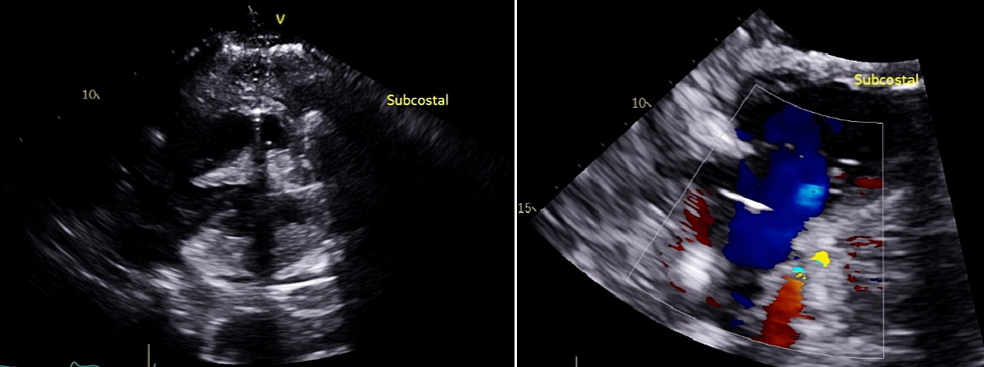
Bedside TTE. V: urgent transthoracic bedside echocardiogram, TTE: transthoracic echocardiogram.
